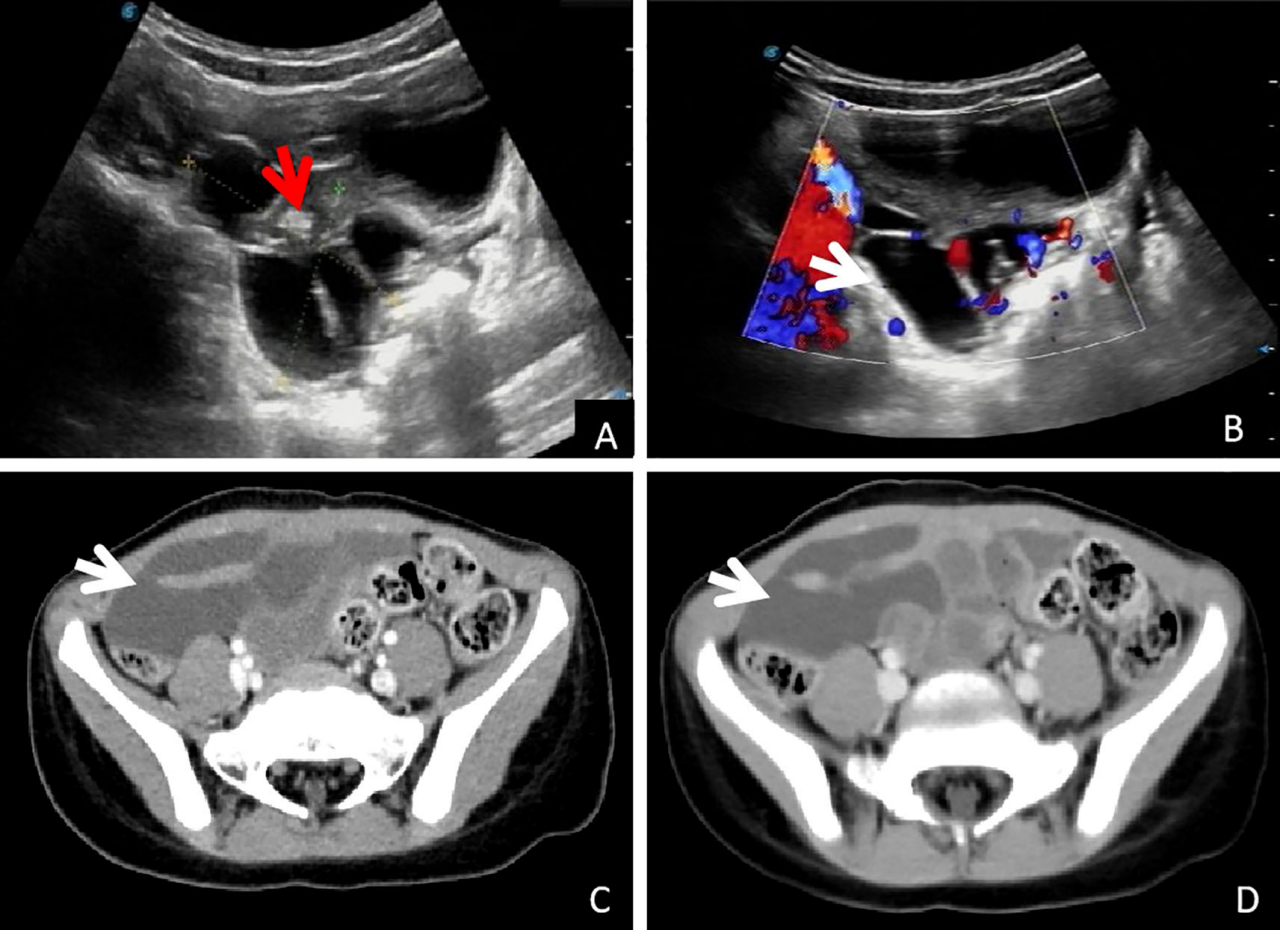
Imaging examination: (A, B): Conventional ultrasound examination showed pelvic cystic occupying, visible separation (white arrow) and wrapped small intestine (red arrow), and blood flow signals with stars on the CDFI septum (white arrow). (C, D): Abdominal enhanced CT showed cystic mass in the right pelvic cavity, and no enhancement was observed in arterial and venous phases (white arrow).
